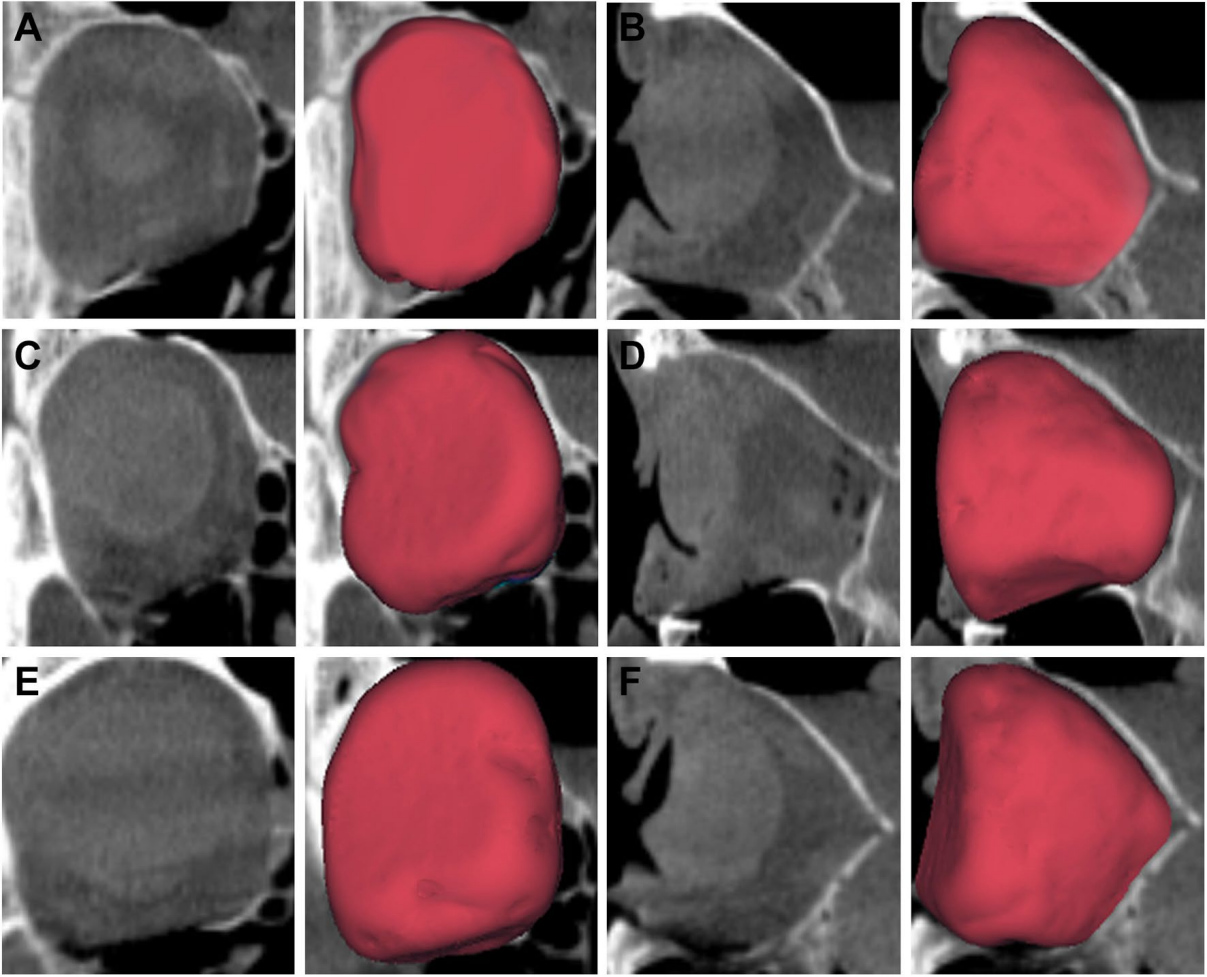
Reconstructed orbits. Cone-beam CT scans of the right orbit in coronal (a, c, d) and sagittal (b, d, e) view. Each orbit was reconstructed using Ethisorb (a, b), marPOR with a strength of 0.85 mm (c, d) and marPOR with a strength of 1.5 mm (e, f). Segmented volumes (in red) were automatically created by specialized software
